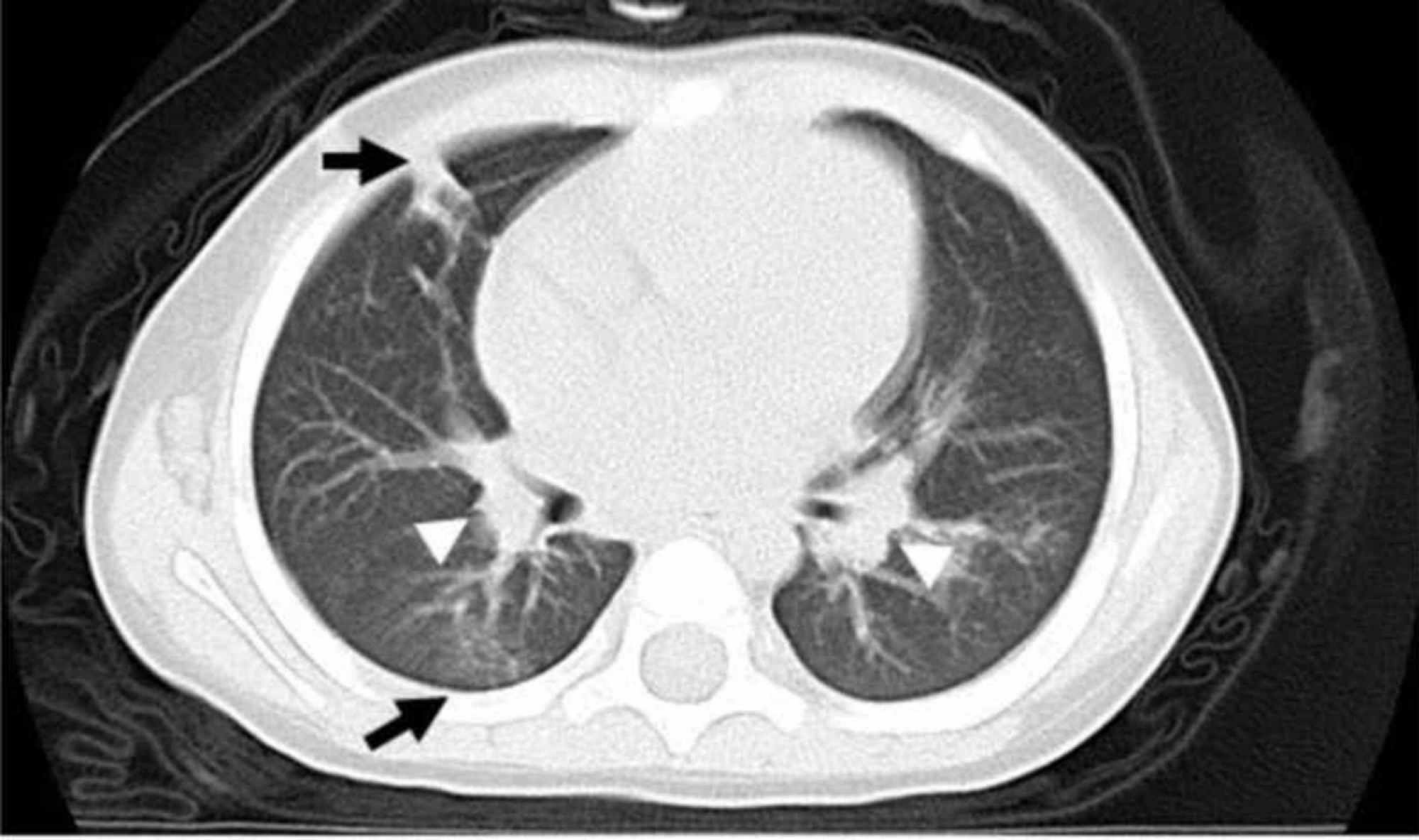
CT Scan of the Lungs Axial image from a scan with lung windows shows faint ground-glass and micronodular opacities in the lower lungs and swelling of both mediastinal lymph nodes (white head arrow). The ground-glass opacities have a marked peribronchovascular distribution in the lower right lung (arrow).
